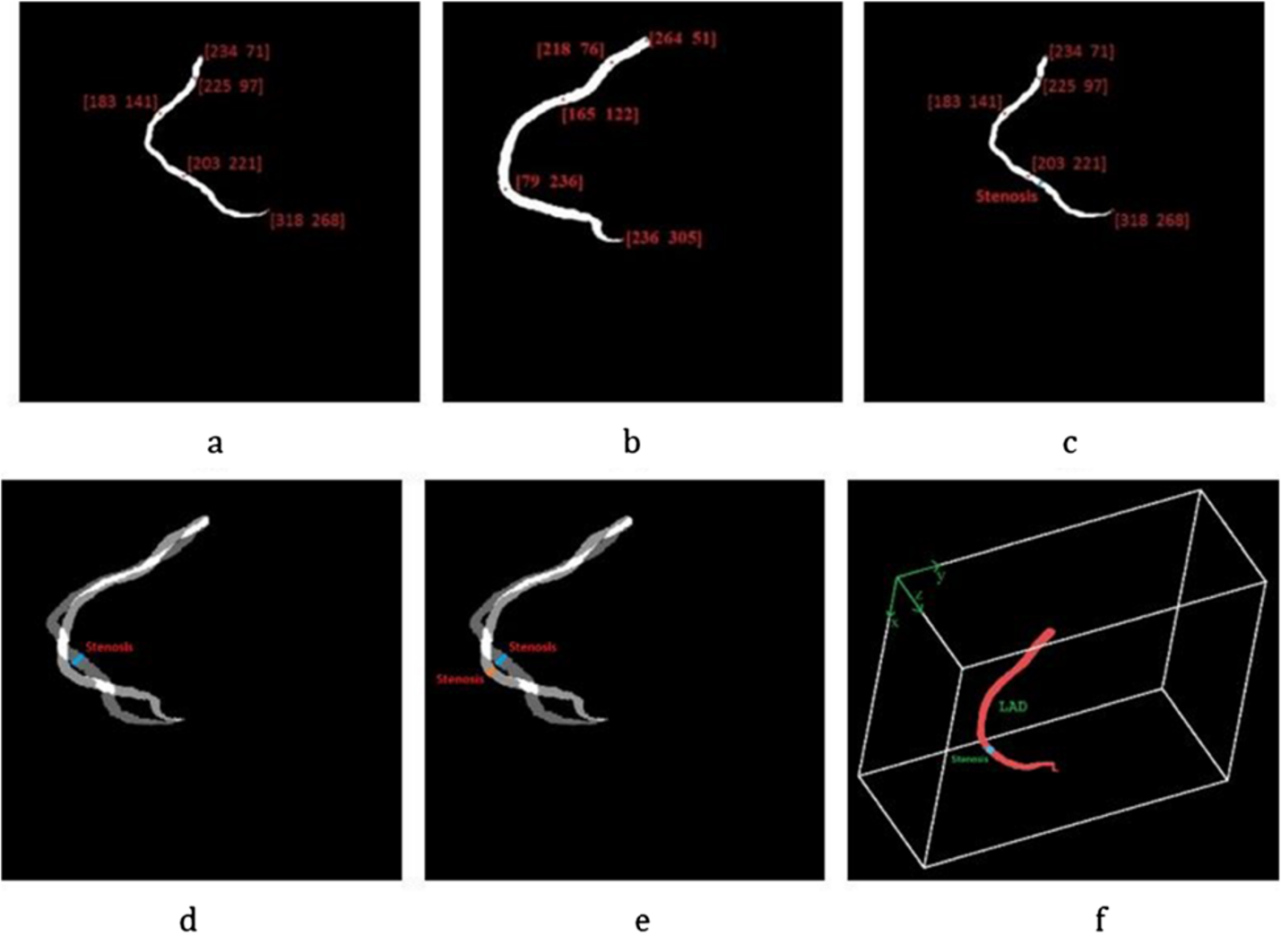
The result of coronary artery registration to locate 3D stenosis point on the CTA. (a) Segmented LAD from angiogram *I*_*A*_ with candidate control points in 2D plane (*u*^'^, *v*^'^). (b) Segmented LAD from CTA with candidate control points, projected on 2D plane (*u*, *v*). (c) Marked stenosis position in the angiogram. (d) Registration result using the proposed method for both modalities shown on plane *I*_*R*_. (e) Stenosis position estimated in the registration plane *I*_*R*_ on coronary artery from CTA. (f) 3D position of stenosis in the original coordinate system (*x*, *y*, *z*).
